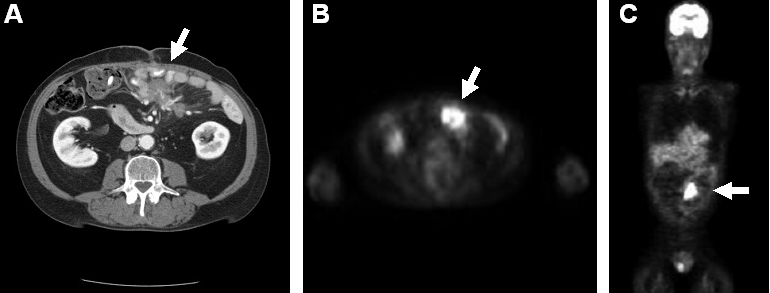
Pre-operative CT and FDG-PET demonstrating the incidentally discovered mesenteric mass. (A) The CT scan shows an ill-defined mass in the anterior aspect of the small bowel mesentery with linear adjacent fat stranding (arrow). (B) FDG-PET scan shows a heterogeneous moderately hypermetabolic focus in the anterior abdomen corresponding to the lesion on the CT scan (arrow). There are no other abnormal hypermetabolic foci. (C) Coronal image of the FDG-PET scan.
